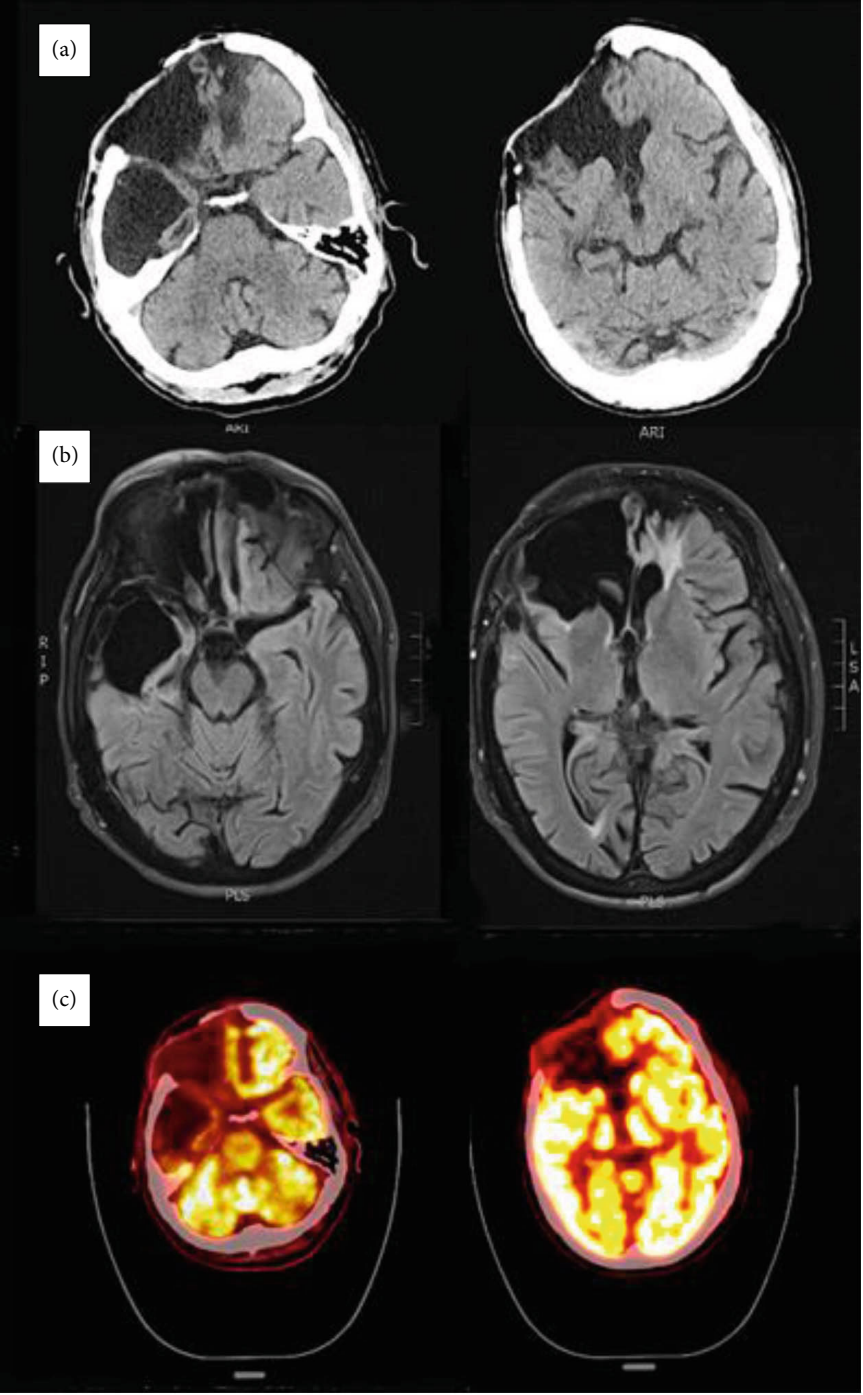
Corresponding axial computerized tomography (CT), magnetic resonance imaging (MRI; fluid-attenuated inversion recovery), and fluorodeoxyglucose positron emission tomography (FDG-PET) scans of the brain. (a) CT shows a large right frontal calvarial vault defect with underlying extensive volume loss/encephalomalacia in the right frontal and anterior right temporal lobes with surrounding gliosis. There is also volume loss and gliosis at the anteroinferior aspect of the left frontal lobe. (b) MRI corroborates the CT findings in a greater detail. (c) FDG-PET shows hypometabolism of right frontal, anterior right temporal, and left anterior inferior frontal lobes corresponding to the CT and MRI findings.
